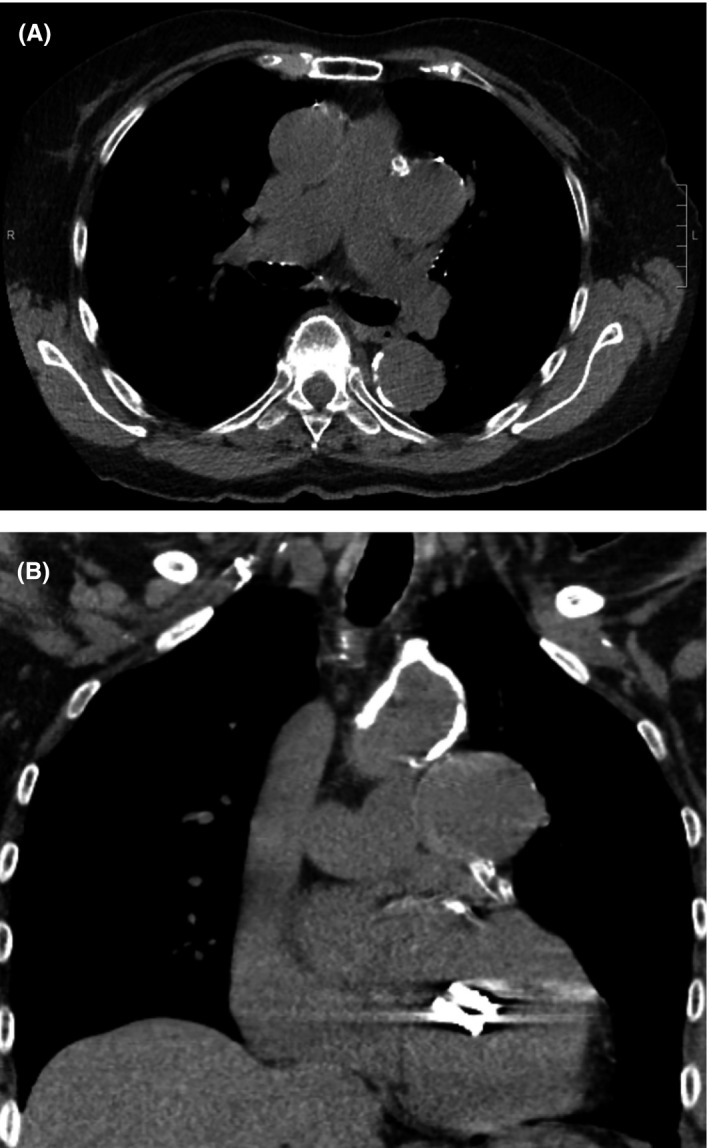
A, Noncontrast CT chest(transverse) showing a 44 × 39 mm aneurysmal dilation of SVG to OM. B: Noncontrast CT chest(coronal) showing a 44 × 39 mm aneurysmal dilation of SVG to OM
